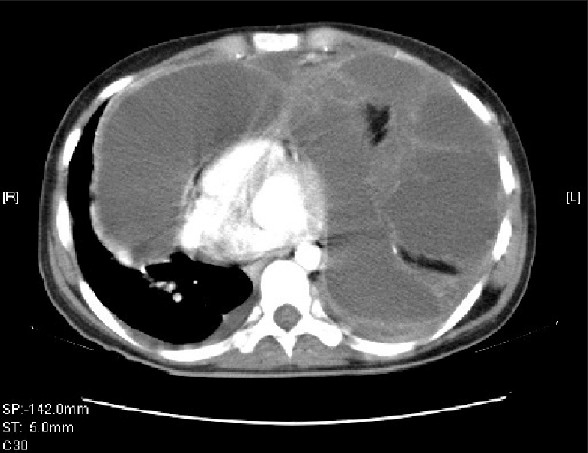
Re-formatted coronal CT image
